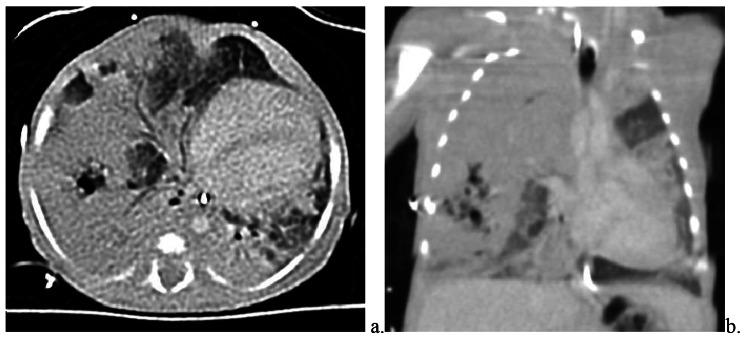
Computed Tomography (CT) Scan with Contrast, Chest. Transverse (a) and Coronal (b) Views
